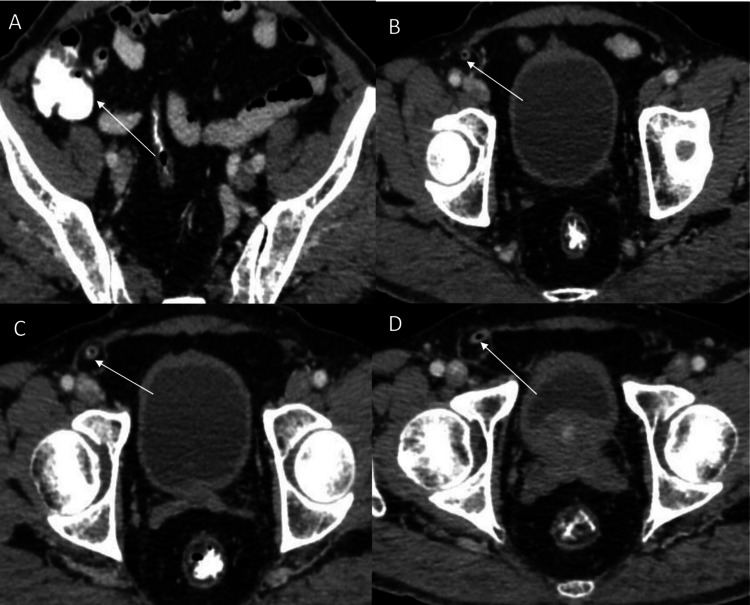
Axial CECT images showing cecum (A, white arrow), with the appendix arising from the cecum entering into the right inguinal canal with the presence of air within (B-D, white arrow) CECT: contrast-enhanced computed tomography
